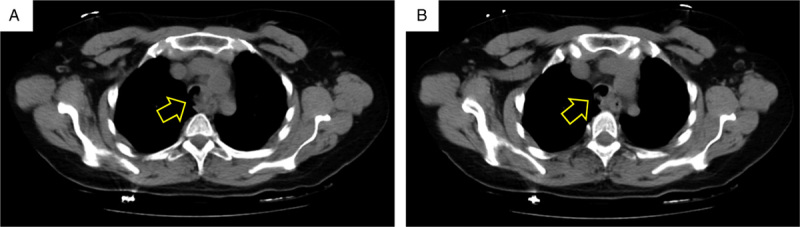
(A and B) A computed tomography scan of the chest showing endotracheal mass lesion narrowing the airway lumen (arrows).
